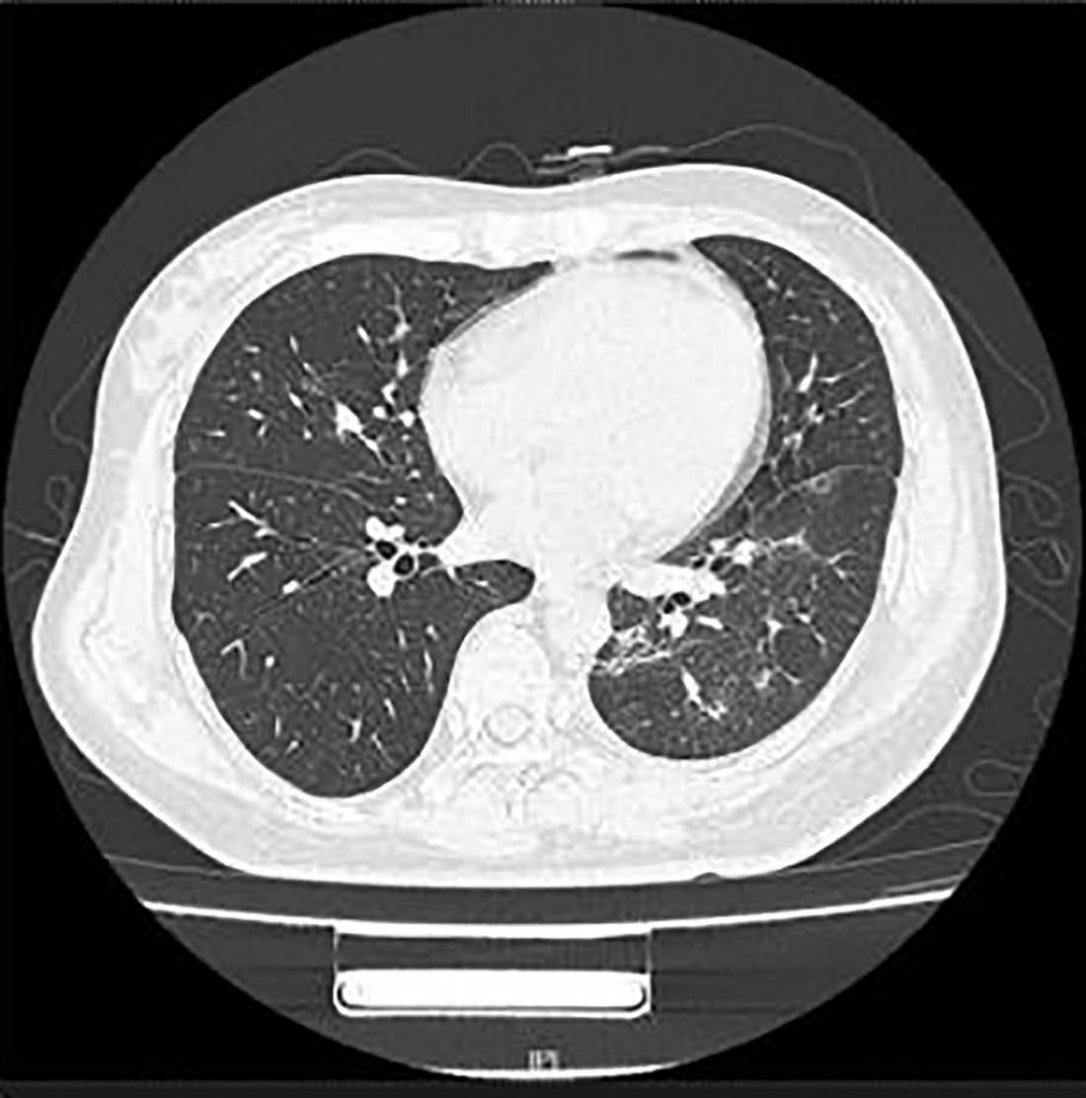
Patient was reexamined using pulmonary CT on October 30, 2023. CT = computed tomography.
